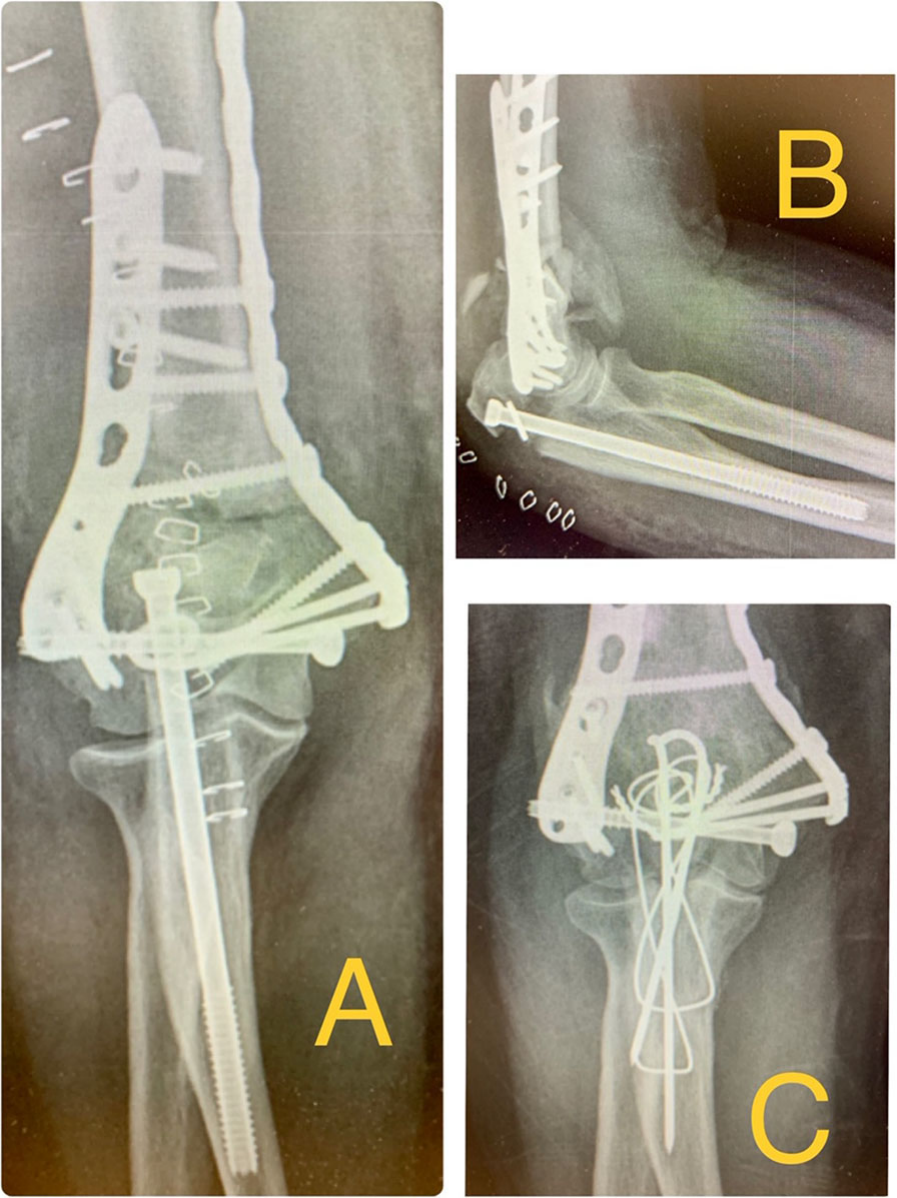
a, b 73 year old man who presented a conminuted supraintercondylear fracture of the humerus. Osteosynthesis of the fracture with two plates and a 6.5 mm thick and 120 mm long screw with washer for the olecranon osteotomy. X-ray on the first postoperative day, with acute failure of osteosynthesis with impaction of the screw into the medullary canal of the ulna. c Control X-ray at 2 months after revision surgery to a tension band cerclage, showing osteotomy consolidation and good functional result
